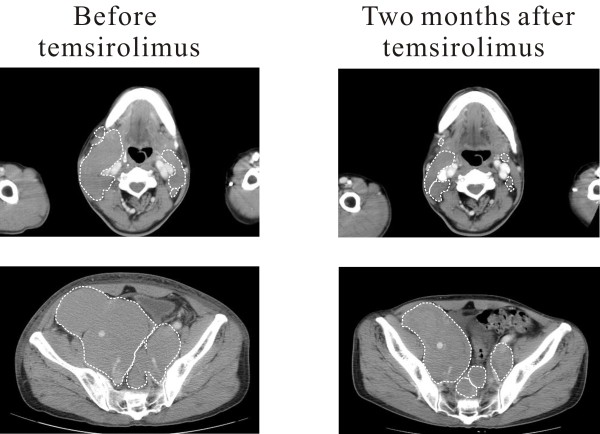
Computed tomography images of MCL. Areas of major lesions (surrounded by white broken lines) significantly regressed after two months of temsirolimus treatment.
